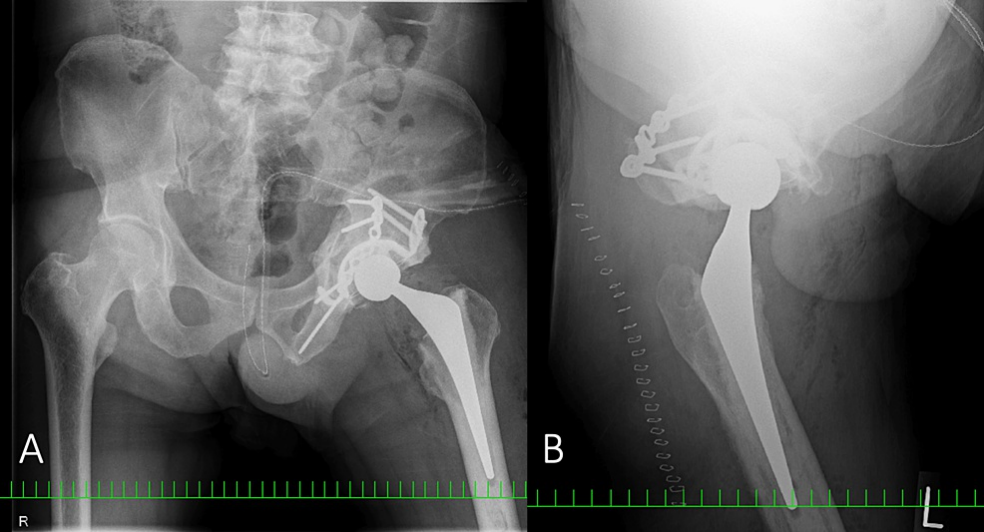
Plain radiograph after the first THA A) Frontal radiograph; B) Lateral radiograph THA - total hip arthroplasty
